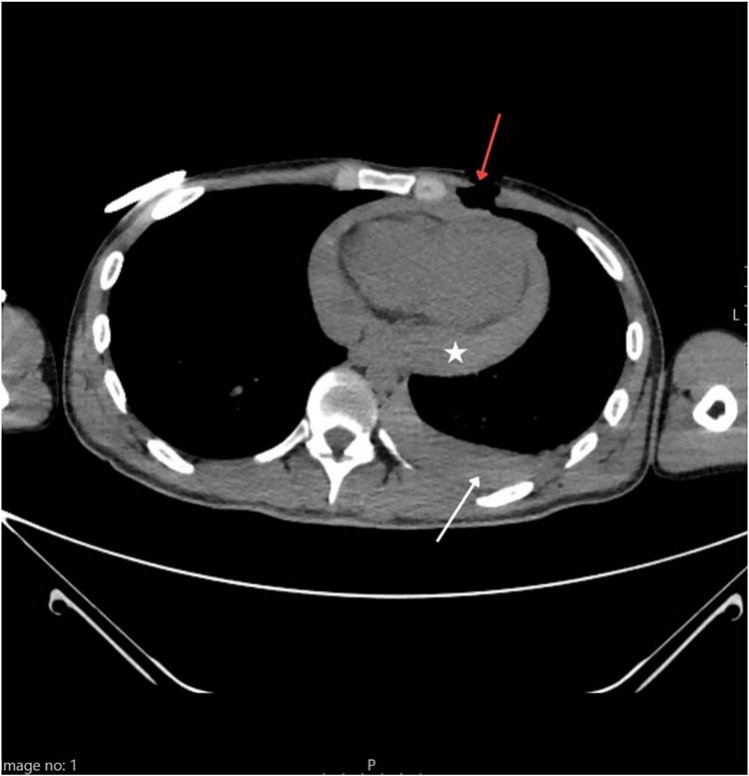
Thoracic CT showing a penetrating wound to the left pectoral area (red arrow) along with hemorrhagic pleural effusion (white arrow) and hemopericardium (star).
